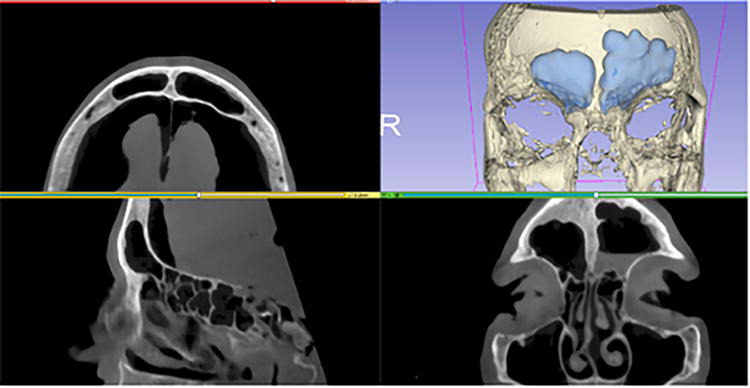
Preoperative CT scans and segmented structures (bone and frontal sinus) on 3D Splicer application. This figure is quoted with permission from Neves et al. (CC BY 4.0).^
